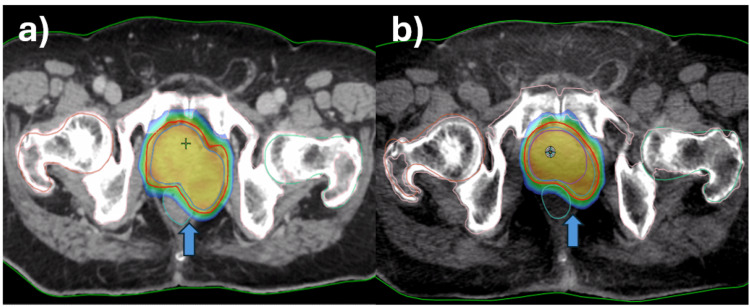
Radiotherapy dose distribution (blue arrow) in (a) planning CT vs (b) last week CBCT CT: computed tomography, CBCT: cone beam computed tomography
